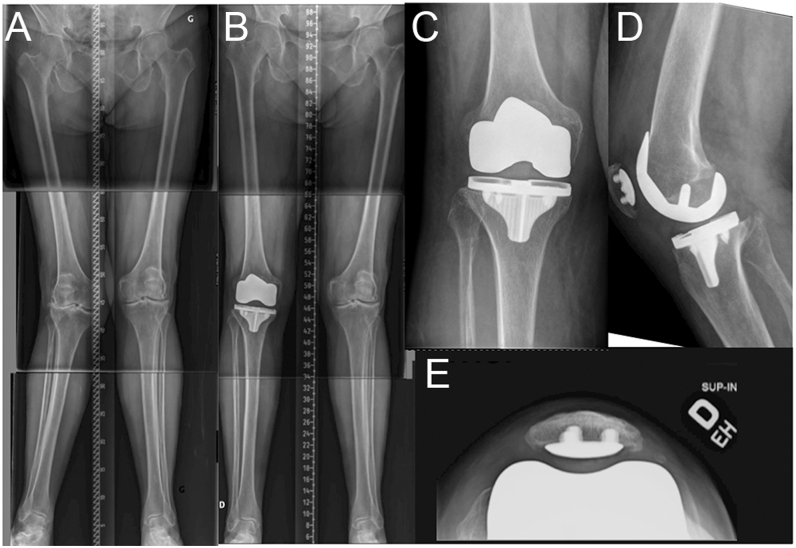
Mild valgus case. (A) Preoperative long-leg radiographs of an 82-year-old female with a mechanical HKA of 17° valgus and an arithmetic HKA of 6° valgus (distal femur 9° valgus and proximal tibia 3° varus). (B, C, D, E) Postoperative radiographs after an uncemented rKA-TKA. According to the rKA protocol, the femur valgus was reduced to 5°, while the tibia was maintained at 3° varus, resulting in a HKA of 2° valgus. The femoral implant was placed without femoral rotation, and the patella was resurfaced. Limited pie-crusting of the posterolateral structures was necessary to obtain ligament balance.
